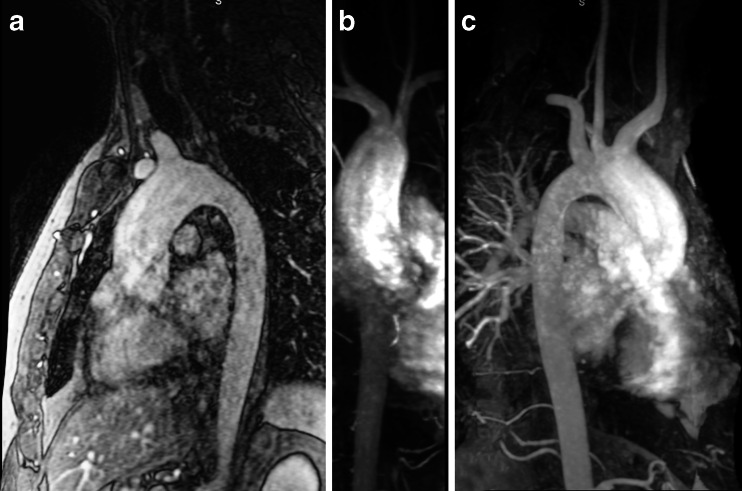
Contrast-enhanced MR angiography of the aorta in a 50-year-old man with a bicuspid aortic valve and moderate stenosis, complicated by mild insufficiency and ascending aorta dilatation. a T1-weighted 3-D spoiled gradient echo after a timed bolus contrast of gadolinium, b-c maximum intensity projection reconstructions
